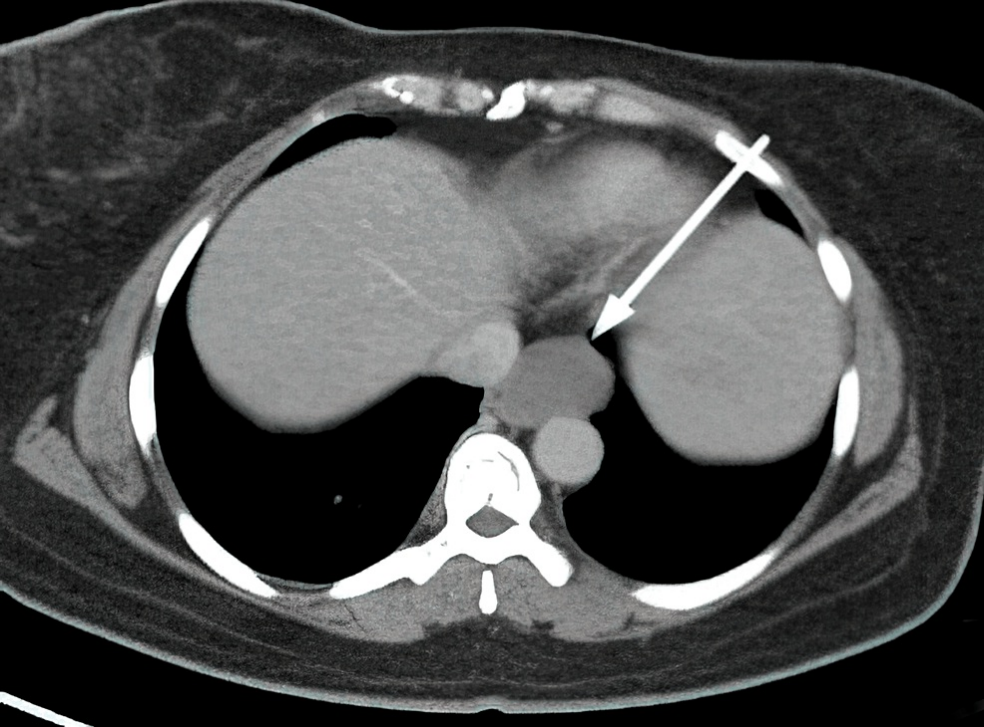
CT lung (transverse view) showing an esophageal mass (white arrow) A well-defined, round, non-enhancing hypodense lesion measuring 2.6 x 2.7 cm in the para-esophageal region on the left side from the middle one-third of the esophagus
